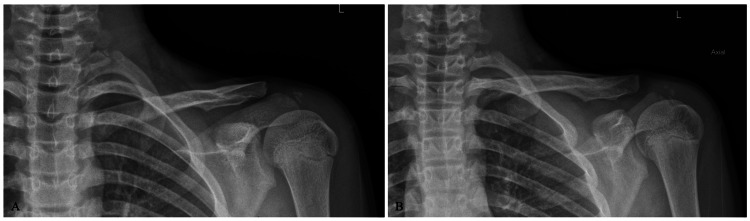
One-year post-injury radiographs, upright anteroposterior and Zanca views (A-B), demonstrating progressive remodeling of the initial segmental clavicle fracture.
